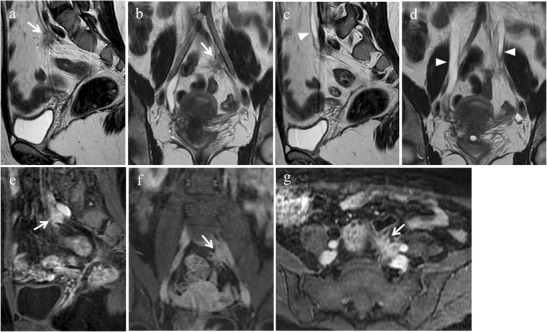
Ureteral endometriosis in a 35-year-old woman with multiple DIE lesions. (a) Sagittal and (b) coronal T2-weighted images show a retroperitoneal solid nodule with spiculated margins (white arrows), with low signal intensity, adjacent to left iliac vessels. (c) Sagittal and (d) coronal T2-weighted images demonstrate dilatation of the ureter upstream and of the contralateral ureter (white arrowheads). On (e) sagittal, (f) coronal and (g) axial contrast-enhanced fat-suppressed T1-weighted images the lesion displays enhancement (white arrows)
